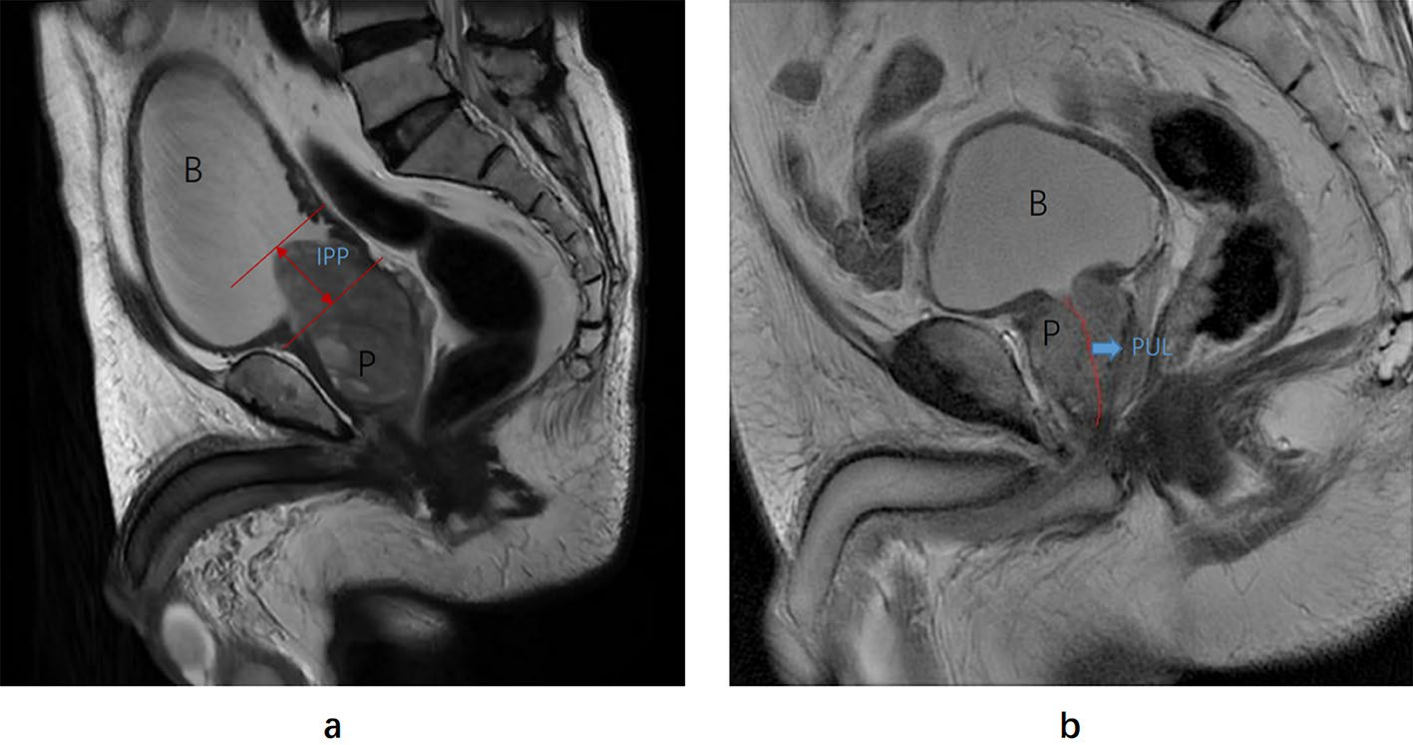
The measurement of the intravesical prostatic protrusion (IPP) and prostatic urethral length (PUL) on T2-weighted sagittal magnetic resonance imaging: a IPP is the vertical length from the protrusion of the prostate (P) to the base of the bladder B. The red line represents the IPP measurement. This patient is 72 years old with an IPP of 25.3 mm. b PUL is the length from the base of the prostate (P) to the apex of the prostate in midsagittal on MRI. The red line represents the PUL measurement. This patient is 68 years old with a PUL of 4.5 cm
